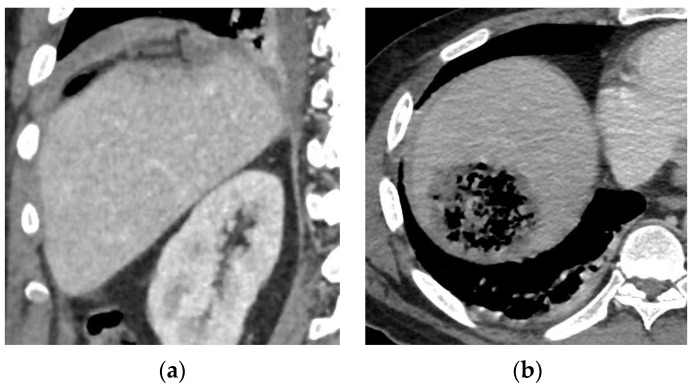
Axial CT scan image after contrast injection showing a poorly limited area containing a little liquid and mainly many gas bubbles, without enhancement wall nor collection (a). Coronal CT scan image after contrast injection showing surgical resection area and the Pezzer drain (b). Hounsfield scale (HU): 60 HU for liver paranchyma and −1000 HU for air.
